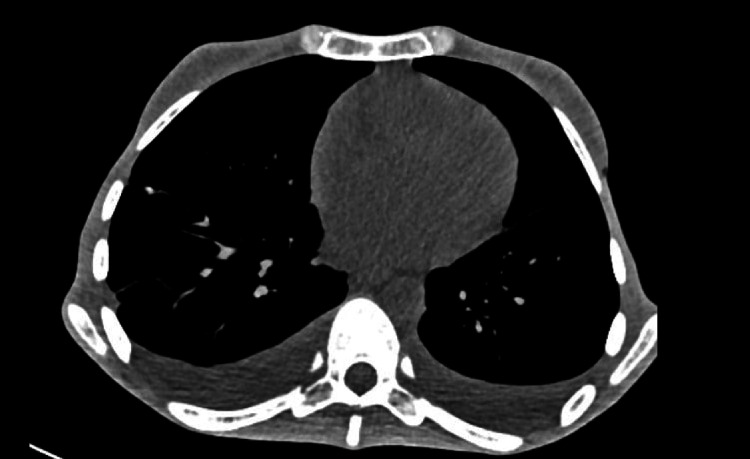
A CT scan of the abdominal organs with bilateral hydrothorax CT: computed tomography
